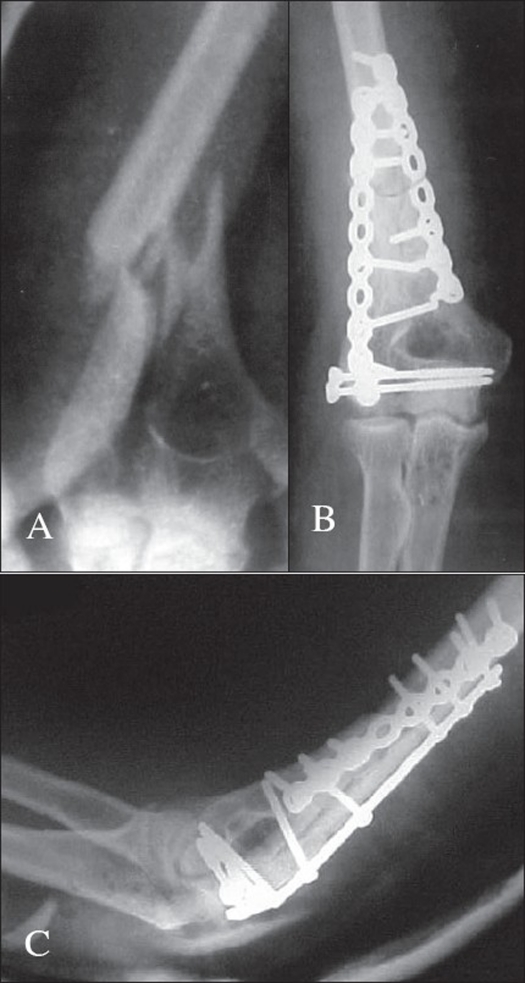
X-ray of elbow A) AP view of pre-operative showing fracture and B) AP view C) Lateral view of post-operative showing fracture union. The olecranon osteotomy is conspicuous by its absence
